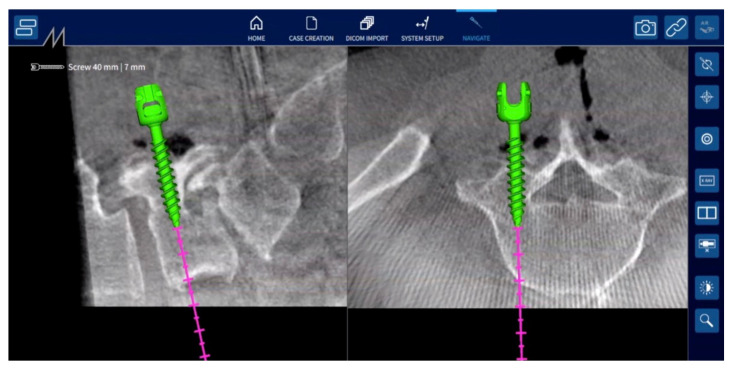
Three-dimensional CT image in NextAR navigation system. During screw insertion procedures, such as pedicle probing, tapping, and screwing, the instrument’s trajectory is displayed on the screen in transverse and sagittal planes of the 3D CT image.
